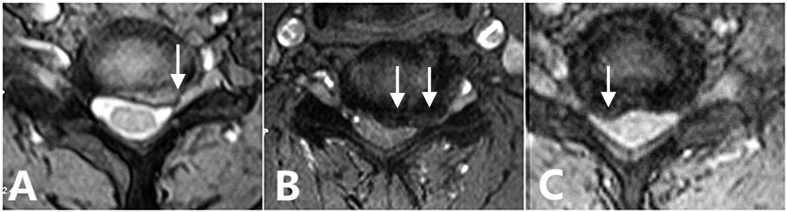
(A) Axial T2-weighted MRI showed ventral nerve root (VNR) compression at the C6–7 intervertebral foramen. (B) Axial T2-weighted MRI showed anterior horn (AH) compression at the paramedial site of spinal canal behind the C6–7 intervertebral level. (C) Axial T2-weighted MRI showed both AH and VNR were compressed at the C5–6 intervertebral level.
